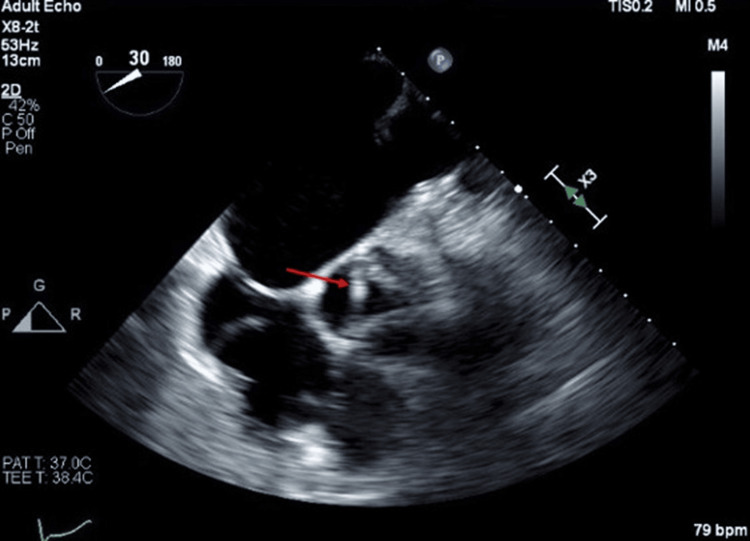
Transesophageal echocardiograph showed multiple filamentous masses on the atrioventricular (AV) leaflets compatible with fibroelastomas denoted by red arrow
